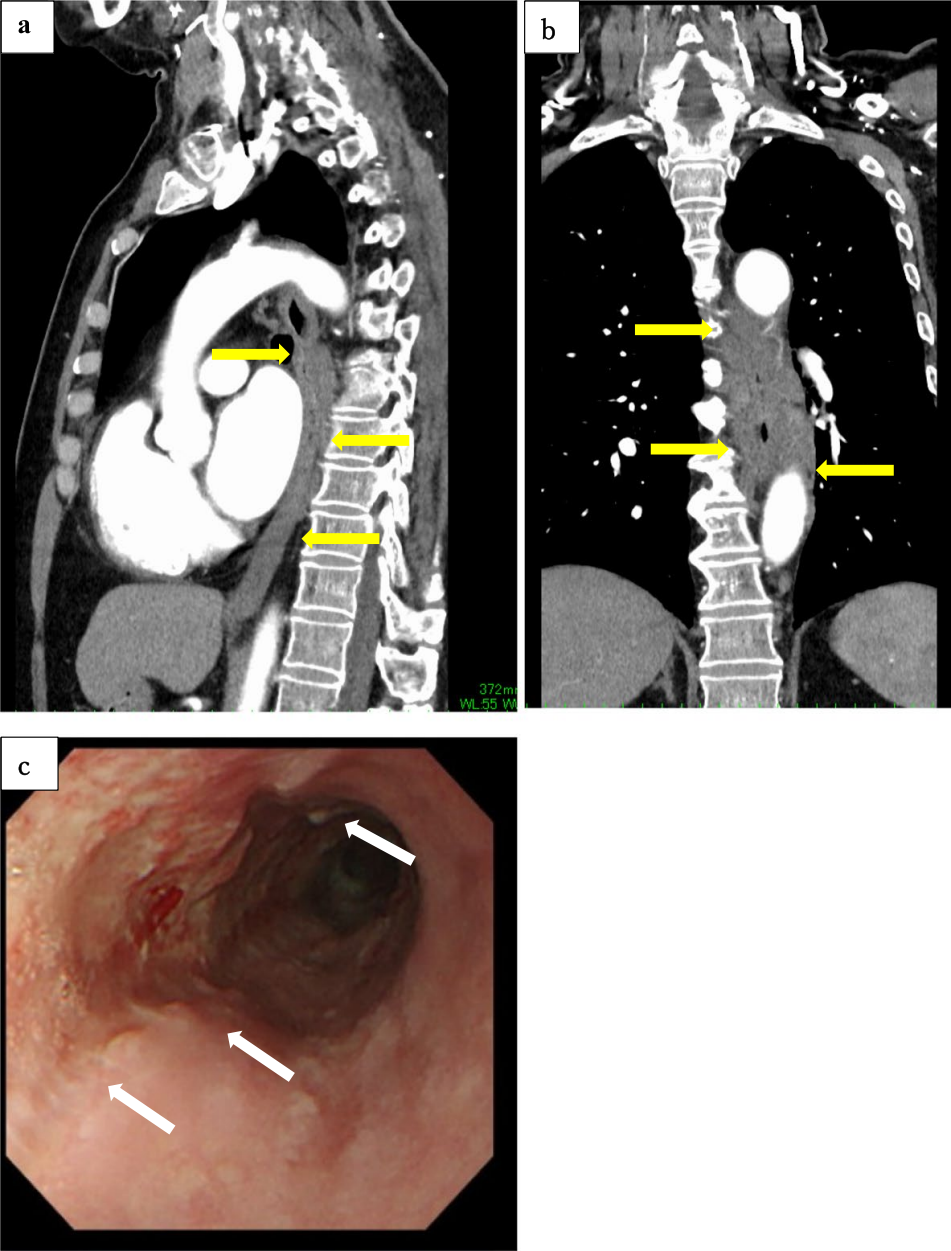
Investigations after transfer to our hospital. a Sagittal, b coronal. CT shows significant improvement in thickening of the esophageal wall. c Endoscopic observation of the ulcer and regenerated epithelium show improvement compared to the previous examination
